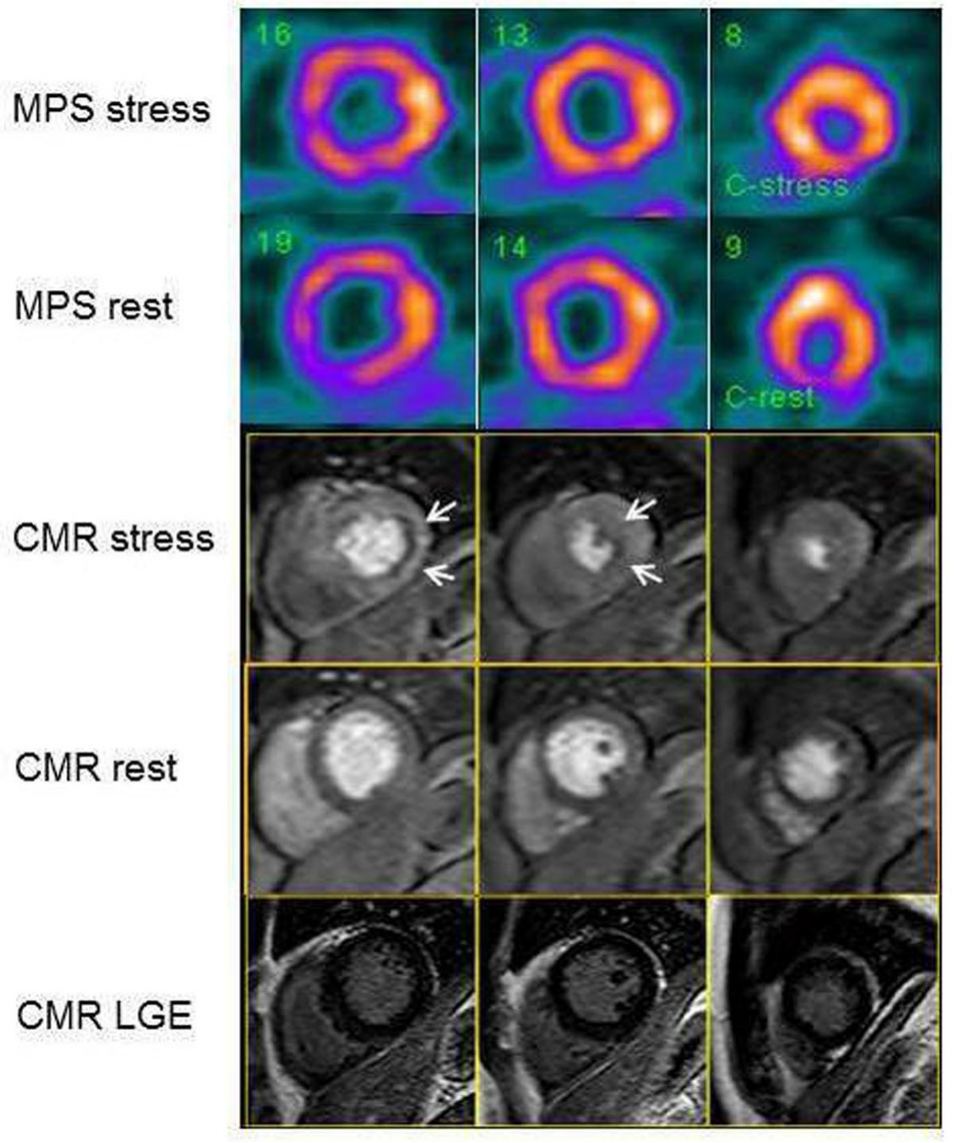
The figure shows short-axis slices of myocardial perfusion at rest and stress with myocardial perfusion SPECT (MPS) and first-pass perfusion at rest and stress with cardiac magnetic resonance (CMR). The MPS and CMR perfusion tracers were injected in the MR scanner during the same adenosine stress session. This is an example of a patient with no stress induced ischemia with MPS. CMR, however, shows stress induced ischemia in the subendocardial portion of the lateral wall (white arrows). CMR late gadolinium enhancement (LGE) images show no signs or myocardial infarction.
